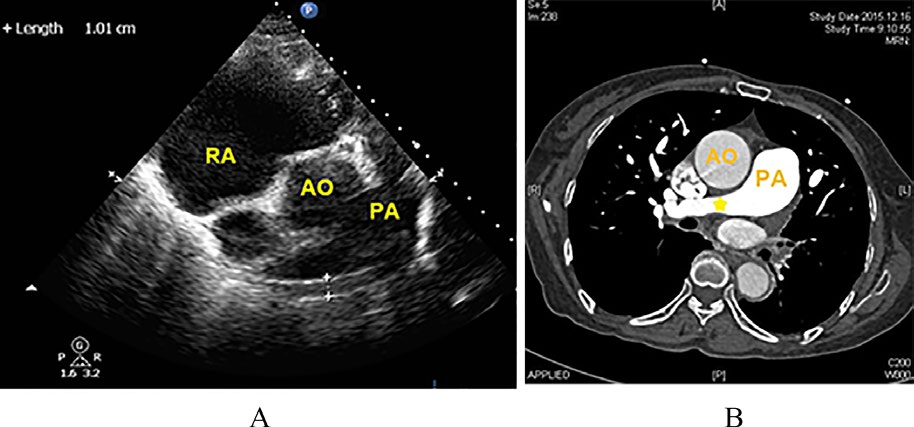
A. Subxiphoid view showed intimal thickening (1cm) in right pulmonary artery and luminal gradual narrowing (movie clip S2). B. CTPA showed right pulmonary artery narrowing (asterisk *). RA = right atrium; AO = aorta; PA = pulmonary artery; CTPA = computed tomographic pulmonary angiography
